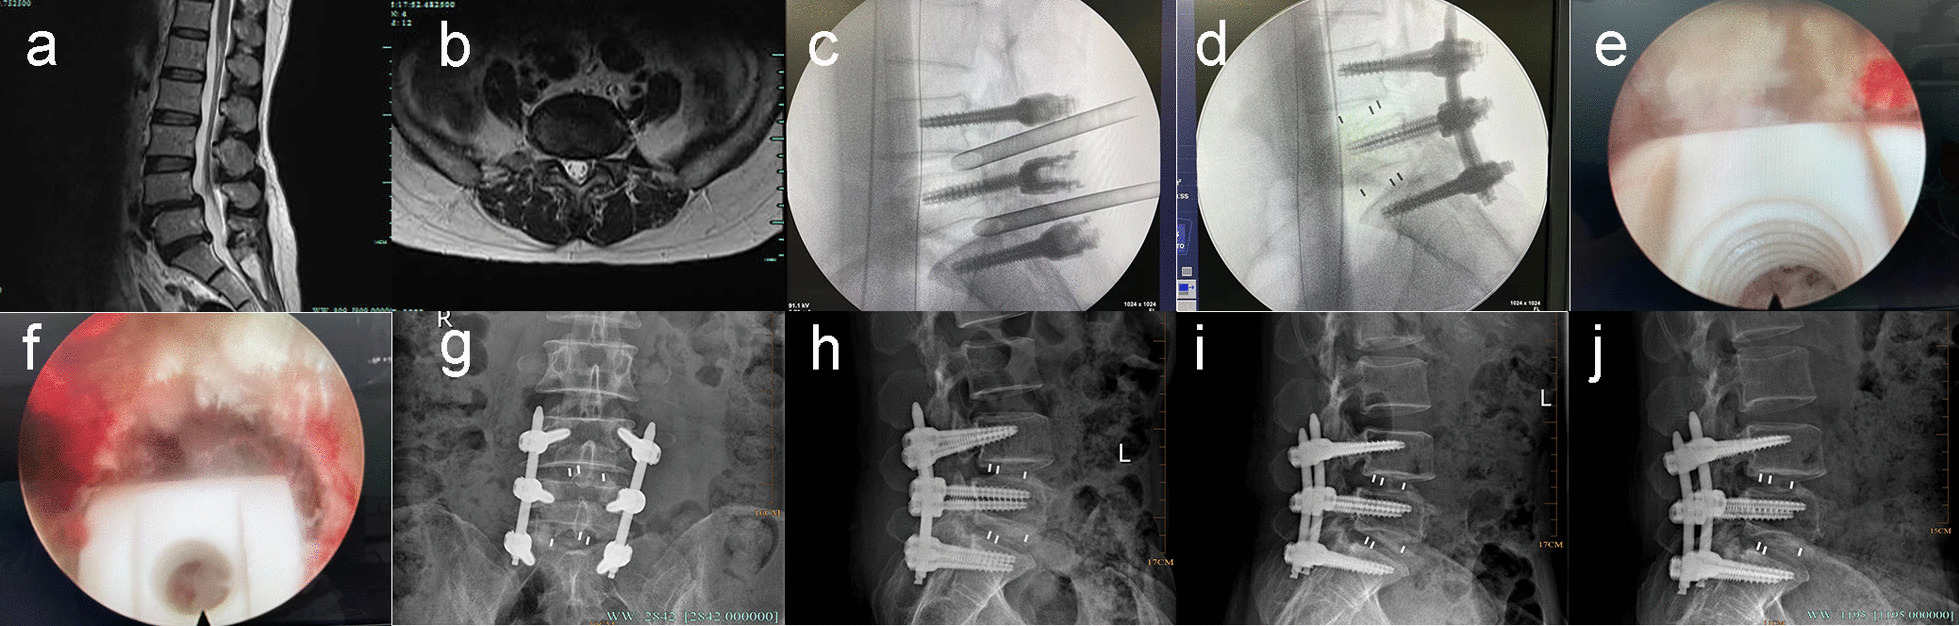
Typical case 4. A 51-year-old female with recurrent low back pain for more than 10 years, aggravating with right lower limb pain and numbness for 3 years. Sagittal MRI shows disc herniation at L5/S1 level and spondylolisthesis at L4/5 level (a). Axial MRI shows disc herniation at L5/S1 level (b). Place the working channel and perform the foraminoplasty (c). Implant the cage (d). Make secondary decompression at L4/5 level (e) and L5/S1 level (f). Anteroposterior and lateral X-ray one month after surgery (g, h). Lateral X-ray three months after surgery (i). Lateral X-ray 1 year after surgery (j)
